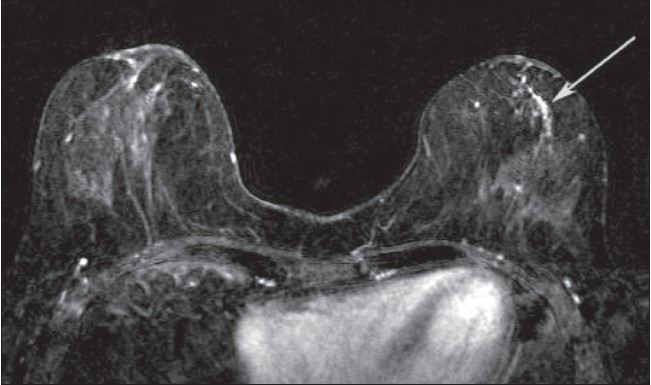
Axial DCE-MRI subtracted image in a patient with a known right breast cancer (not seen at this level) and unsuspected abnormal left breast ductal enhancement (arrow). The lesion on the left was proven to be ductal carcinoma in situe (DCIS) on MRI-guided breast biopsy
